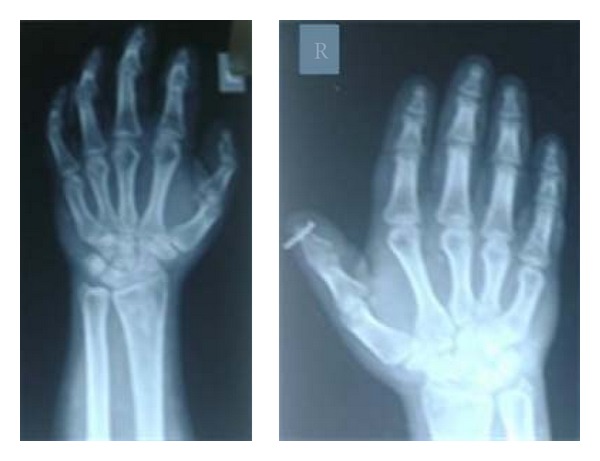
X-ray of hand showing short 3rd, 4th, and 5th metacarpals.
